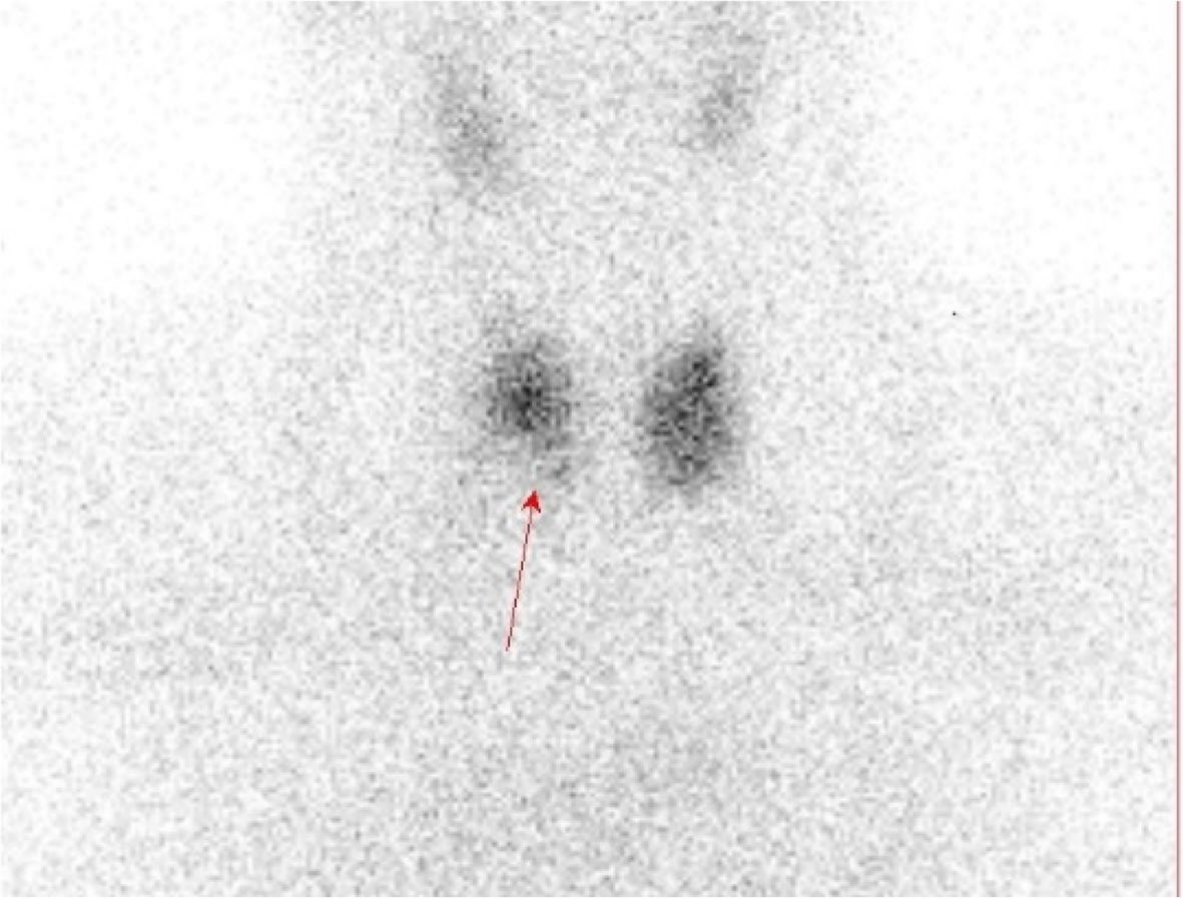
Thyroid ^99m^Tc pertechnetate scintigraphy showing cold area in the right thyroid lobe indicated by arrow
